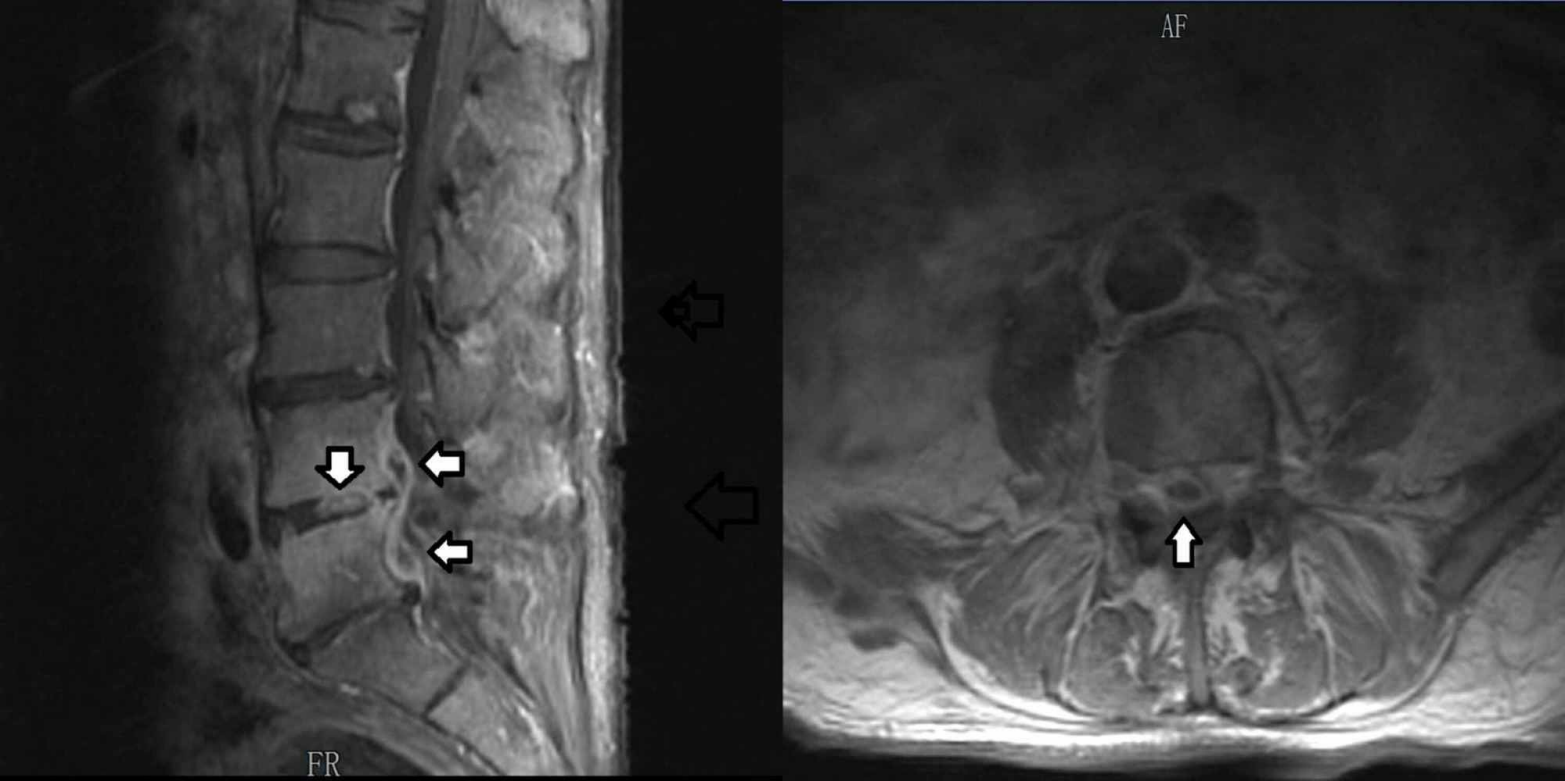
MRI of the L-spine Coronal view (left) and transverse view (right) of the spinal epidural abscess.
